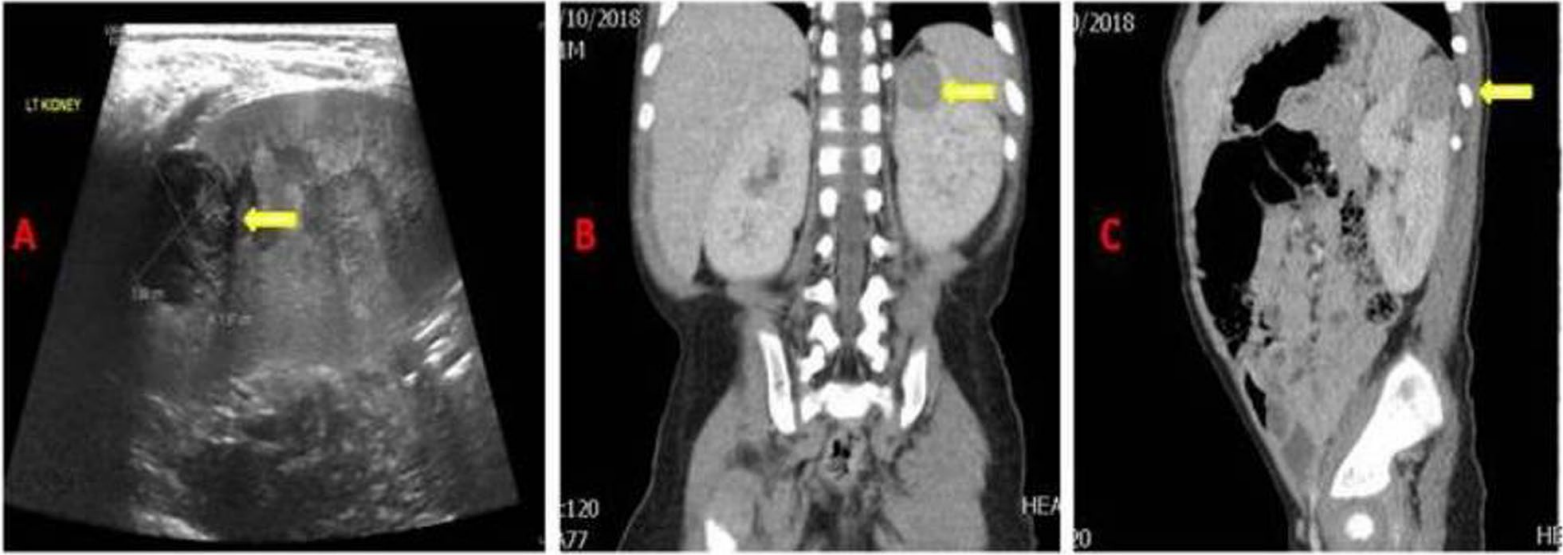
Radiological evaluation. A Renal ultrasound documented a sharply marginated peripheral hypoechoic hypovascular mass at the upper pole of the left kidney measuring 2 × 2 cm. B, C Computerized tomography scan of the abdomen showing a partially exophytic homogenously enhancing mass at the upper pole of the left kidney measuring 1.8 × 1.9 × 2 cm
